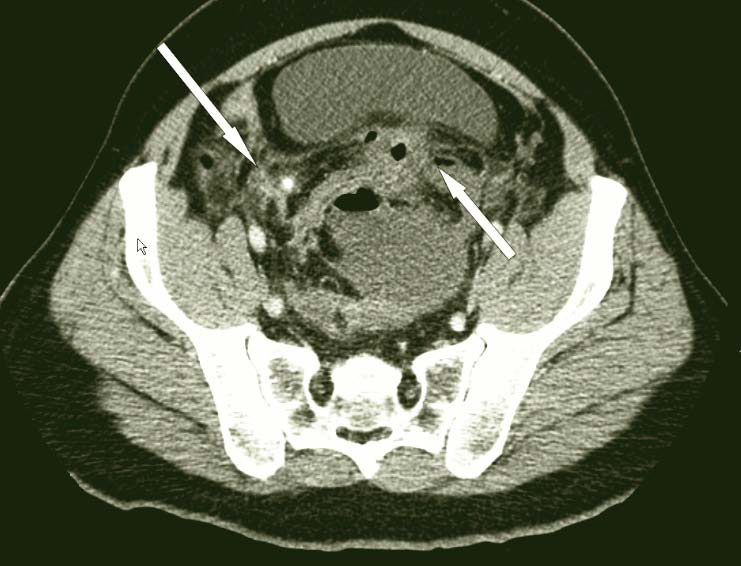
CT Scan showing a necrotic appendix with a stercolith (long arrow) and anterior wall perforation (short arrow).
